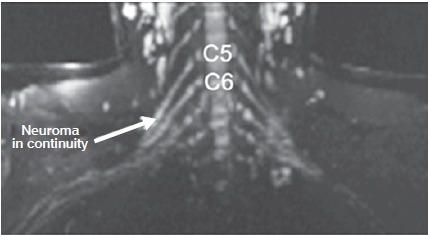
Magnetic resonance imaging, patient 2. Diffusion-weighted neurography - reconstruction in the coronal plane: Thickening and increased signal in the C5 and C6 roots extending to the upper trunk, corresponding to lesion in continuity.
